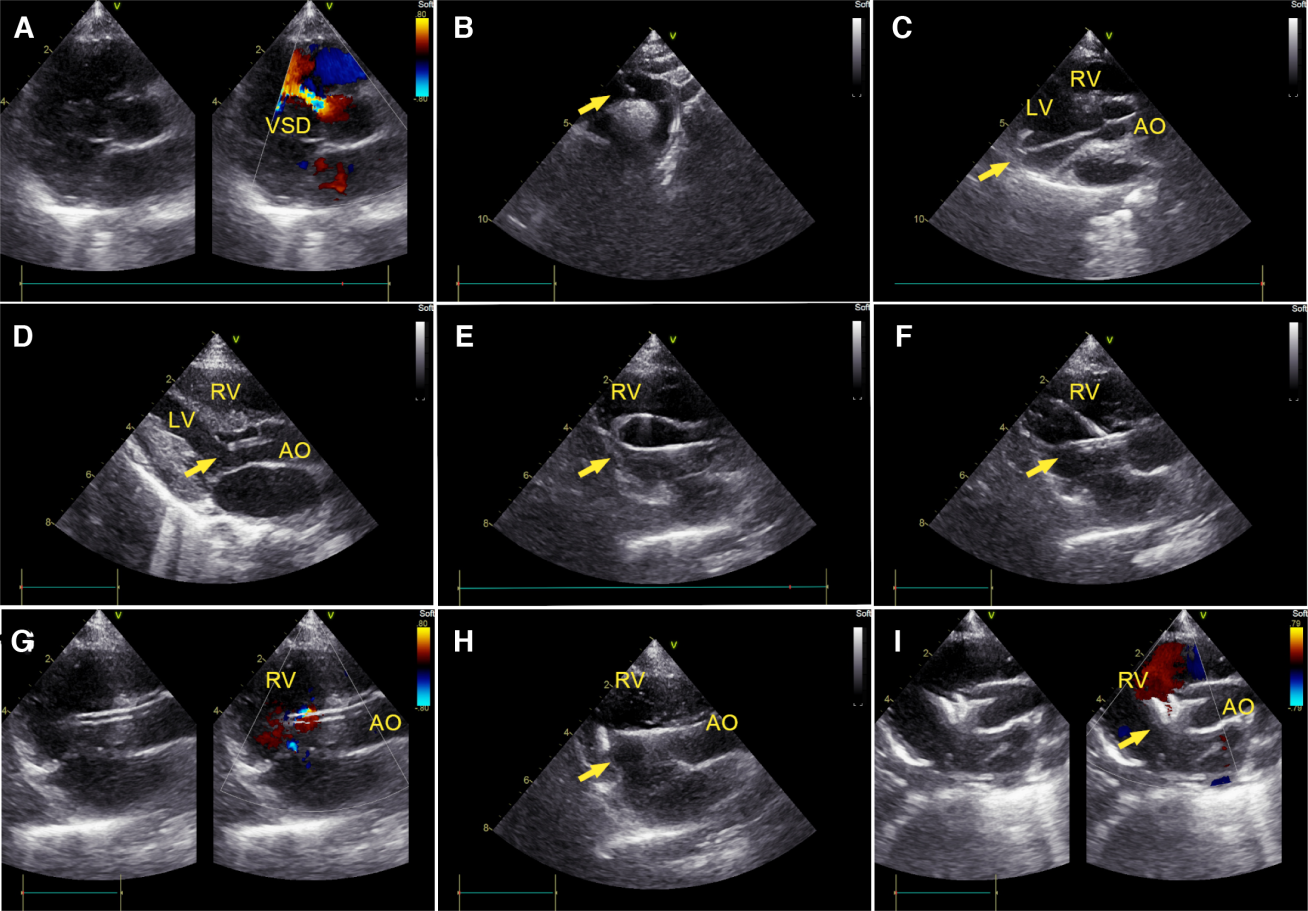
Percutaneous ultrasound-guided perimembranous ventricular septal defect (PmVSD) closure. (A) Image of PmVSD in the short- axis view. (B) A 5-French trimmed pigtail catheter was introduced along a 0.035-inch angled hydrophilic guidewire (Radifocus Guidewire M, Terumo Medical Corporation, Tokyo, Japan) into the descending aorta, then advanced across the aortic arch in the suprasternal aortic arch long-axis view of TTE. (C) Using the long-axis view of the LV, the guidewire crossed the aortic valve, then the pigtail catheter would be advanced into the left ventricle (LV). (D) The cut-pigtail catheter was pulled to the level of the PmVSD, and its trimmed tip was rotated toward the ventricular septum in the LV long axis or the apical five-chamber view. (E) After the pigtail catheter was advanced to the right ventricle (RV) along the hydrophilic guidewire, a 260 cm exchange J-tip guidewire (Cordis Corporation, Miami Lakes, Florida, USA) was advanced along the catheter into the RV. Its soft tip (arrow) was confirmed in the parasternal long axis view of the RV inflow tract. (F) The long delivery sheath (arrow) was inserted over the exchange J-tip guidewire into the RV. (G) The guidewire was pulled back and the “double track sign” was viewed. The tip of sheath was confirmed in the RV. (H) The occluder was placed inside the delivery sheath, and its right disc (arrow) was deployed. (I) The PmVSD was closed after both discs of device were deployed. LV, left ventricle; RV, right ventricle; AO, ascending aorta.
